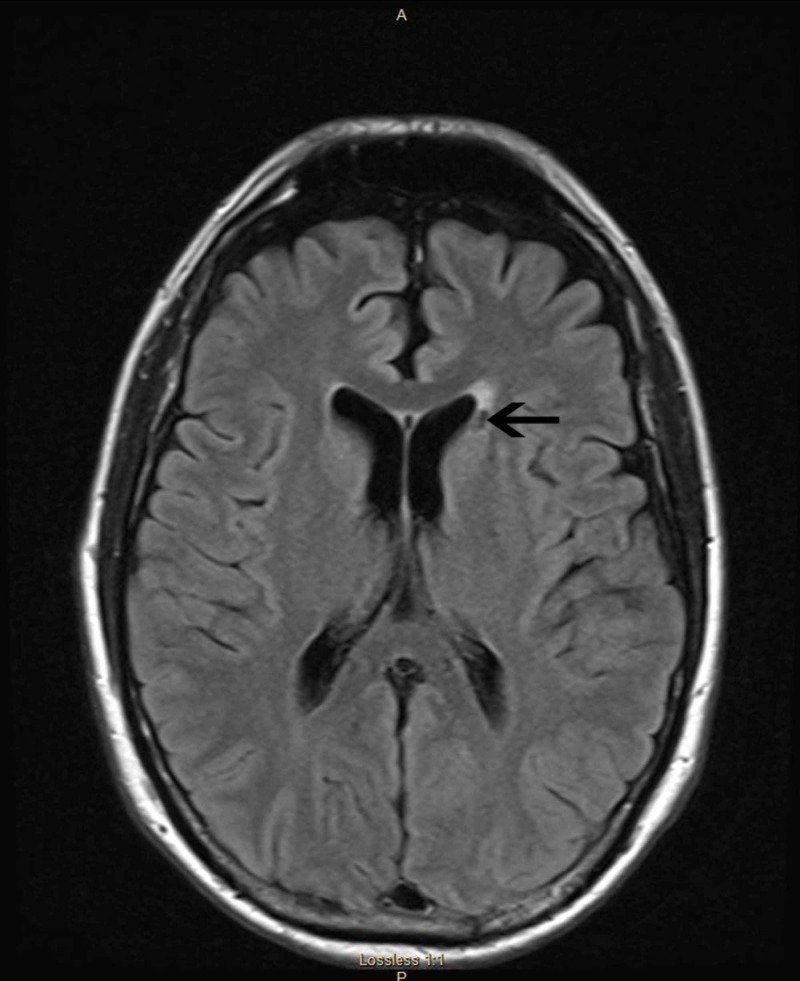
MRI of brain patient 2 MRI of the brain FLAIR sequence shows no acute abnormalities but evidence of a lacunar stroke in the left, just above the head of the caudate nuclei.
